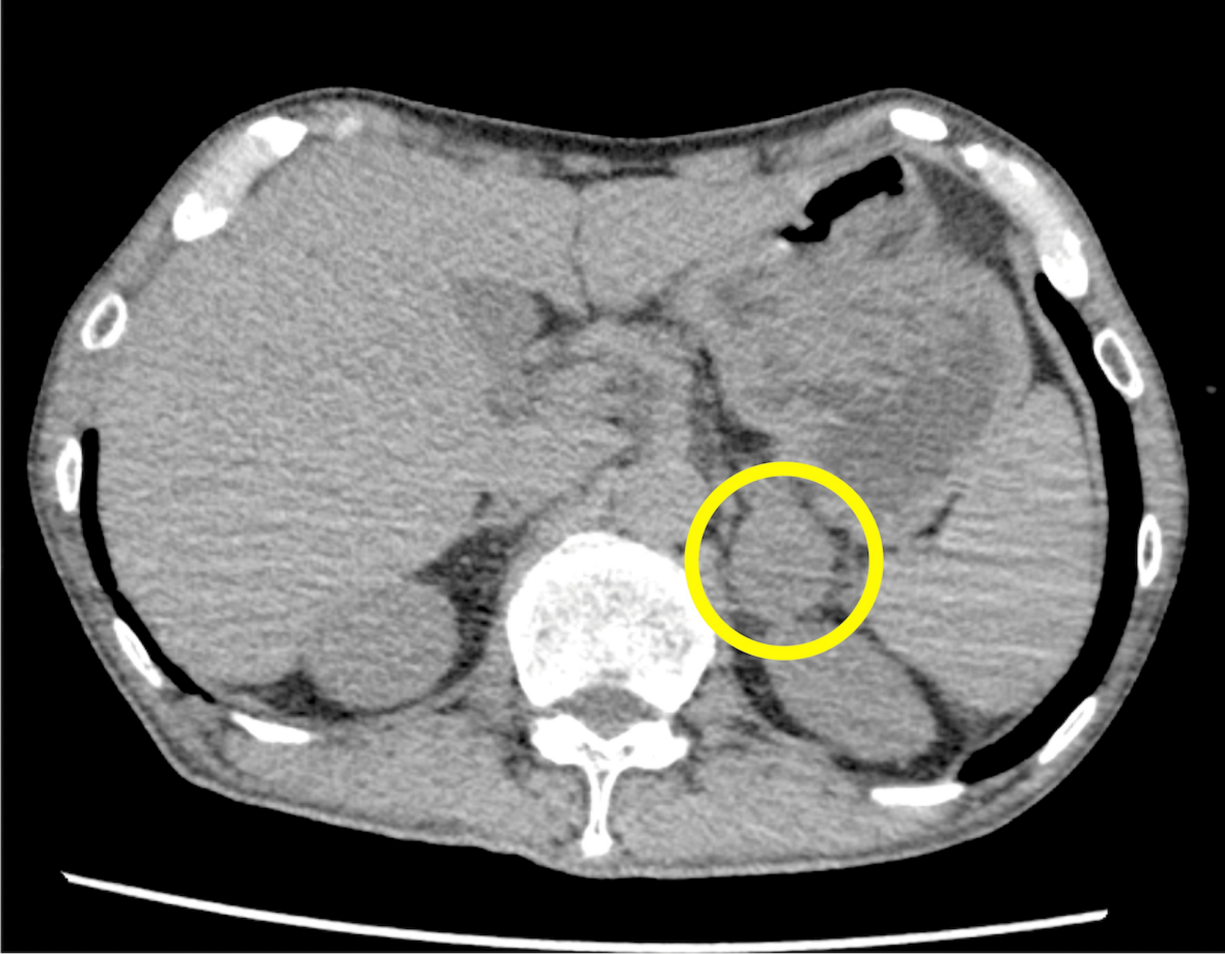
Whole-body computed tomography A left adrenal incidentaloma is observed (yellow circle).
